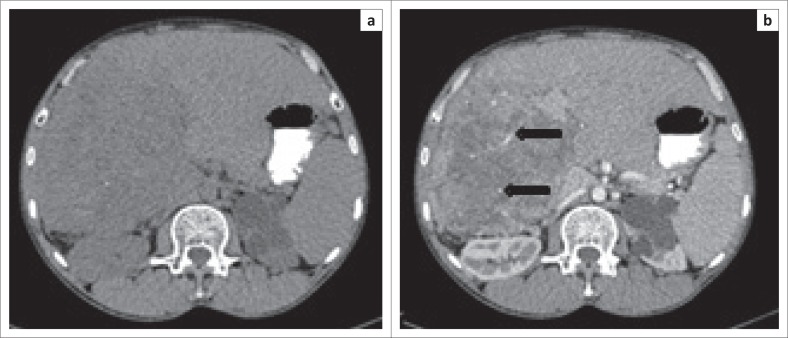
A 73-year-old man who presented with abdominal pain. Axial computed tomography scan images: (a) precontrast; (b) arterial phase shows abnormal internal vessels (black arrows).
